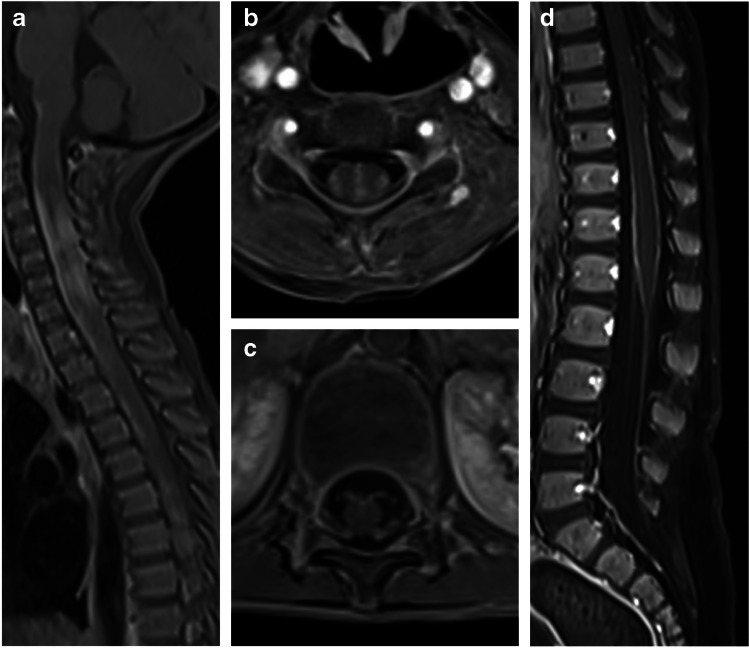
Patterns of enhancement in myelin oligodendrocyte glycoprotein antibody-associated transverse myelitis, on fat-suppressed contrast-enhanced T1-weighted magnetic resonance images obtained from a 5-year-old girl (a), a 4-year-old boy (b, c), and a 21-month-old girl (d). a Sagittal image shows patchy intra-medullary enhancement along the thickened cervical and upper thoracic cord. b, c Axial images show intra-medullary and nerve root enhancement at cervical level (b), and lumbar nerve root enhancement surrounding the conus (c). d Sagittal image shows leptomeningeal enhancement along the lower thoracic and lumbar cord
